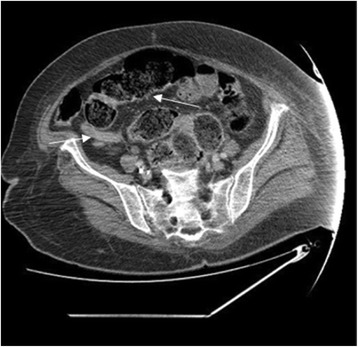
Abdominal CT scan showing fecal impaction in dilated ascending and transverse colon associated with retrocecal appendicitis and pericolic fat stranding (*arrows*)
